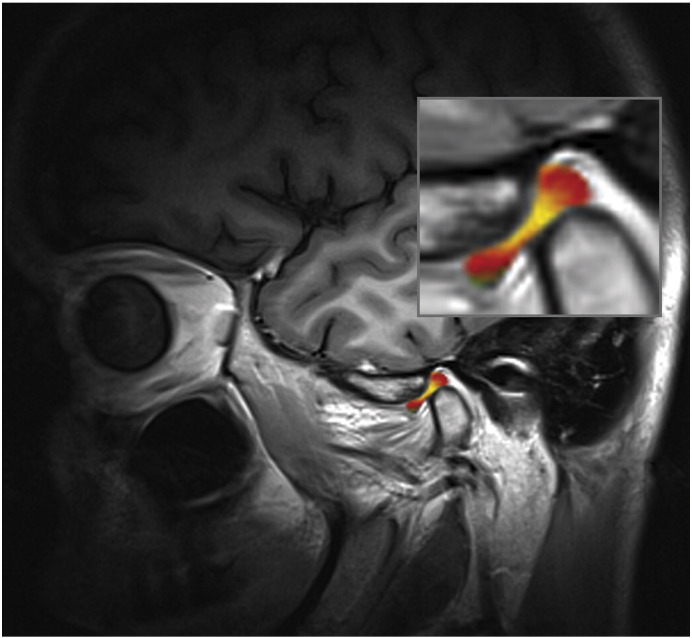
Overlay of T1-weighted image with pseudo-colored IR T1 maps of the TMJ in an asymptomatic volunteer. The TMJ is zoomed for better delineation of the anterior, central, and posterior parts of the TMJ. The central part of the TMJ demonstrates a lower GAG content.
